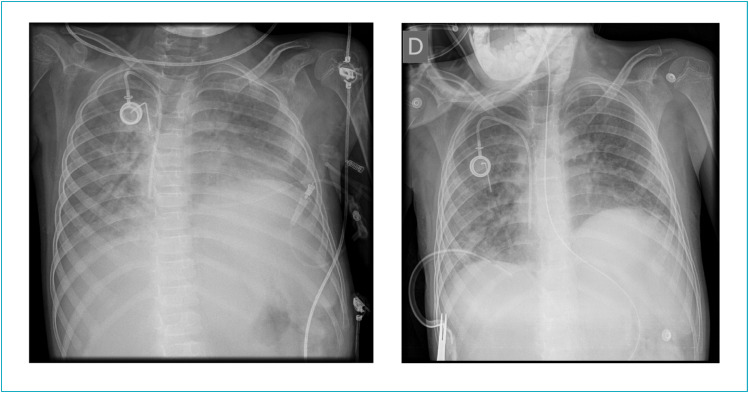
Chest x-ray performed on the first day and 24 hours after suspending dornase alfa therapy in patient A.
